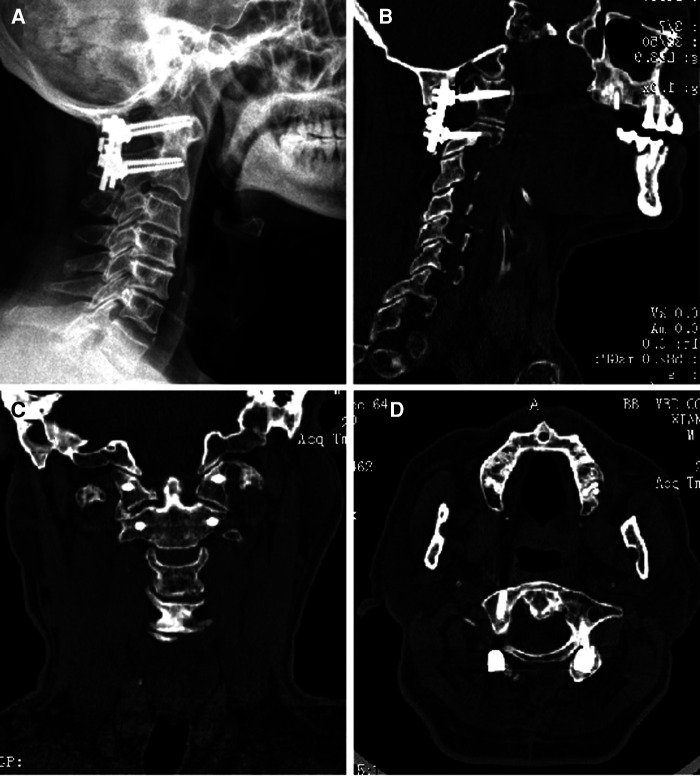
Postoperative x-ray and CT imaging data.
